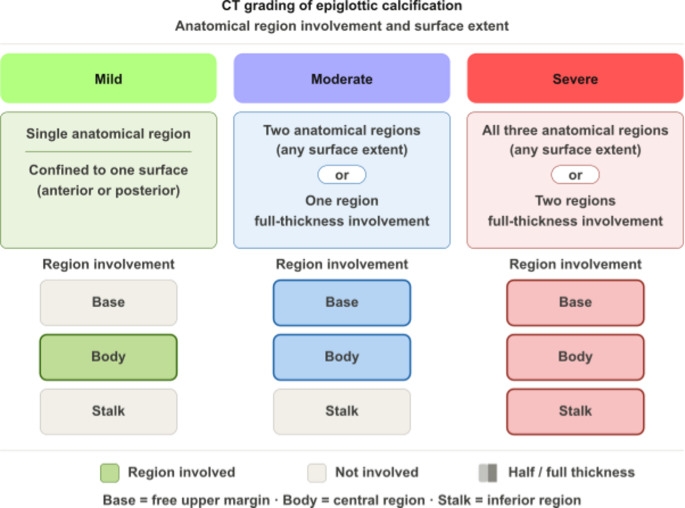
Semi-quantitative CT grading system for epiglottic cartilage calcification. The epiglottis is divided into three anatomical regions: the Base (free upper margin, posterior to the tongue base), the Body (central region at the level of the hyoepiglottic ligament insertion), and the Stalk (inferior region at the thyroepiglottic ligament insertion). Calcification is graded by the number of regions involved and the surface extent of involvement within each region. Mild: calcification limited to a single region, confined to one surface (anterior or posterior). Moderate: calcification involving two anatomical regions (any surface extent), or one region with full-thickness (transmural) involvement. Severe: calcification affecting all three anatomical regions (any surface extent), or two regions with full-thickness involvement. Coloured zones indicate involved regions (green: mild; blue: moderate; dark red: severe); grey zones indicate no involvement. The present case, with almost complete mineralisation of the petiolus with both infrahyoid and suprahyoid portions involved, would exceed the severe grade as defined for this system. After Malkidou N, Fiska A. Anat Cell Biol. 2026;59:54–58
